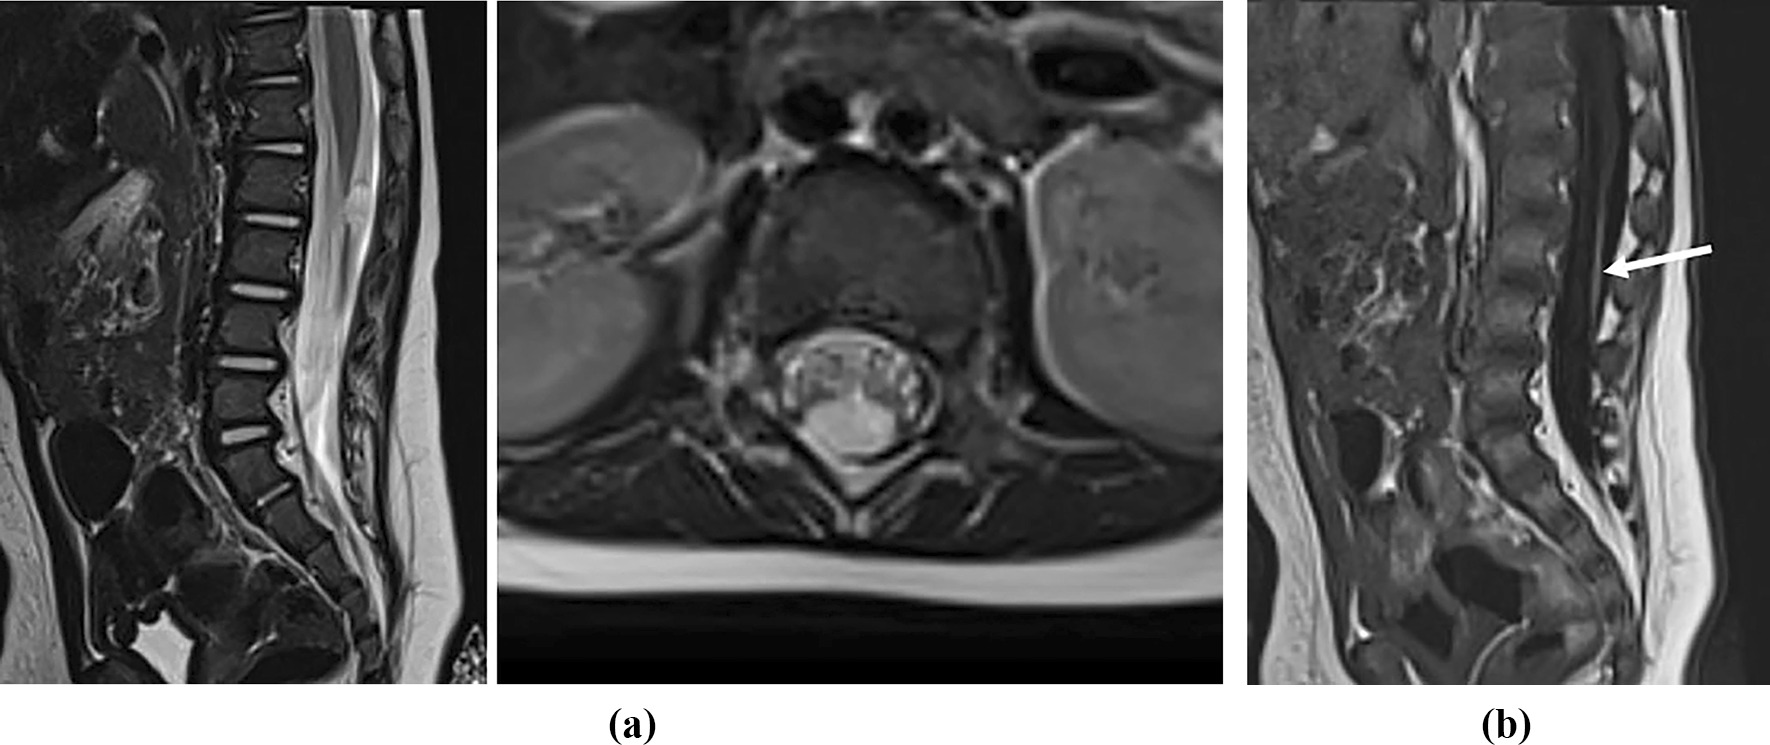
A Midsagittal and axial T2-W magnetic resonance image of lumbar spine at 6 months of age visualizes the persistent conofilar cyst. B Midsagittal T1-W magnetic resonance image at 6 months of age demonstrates a thick filum with fibrolipoma (arrow)
